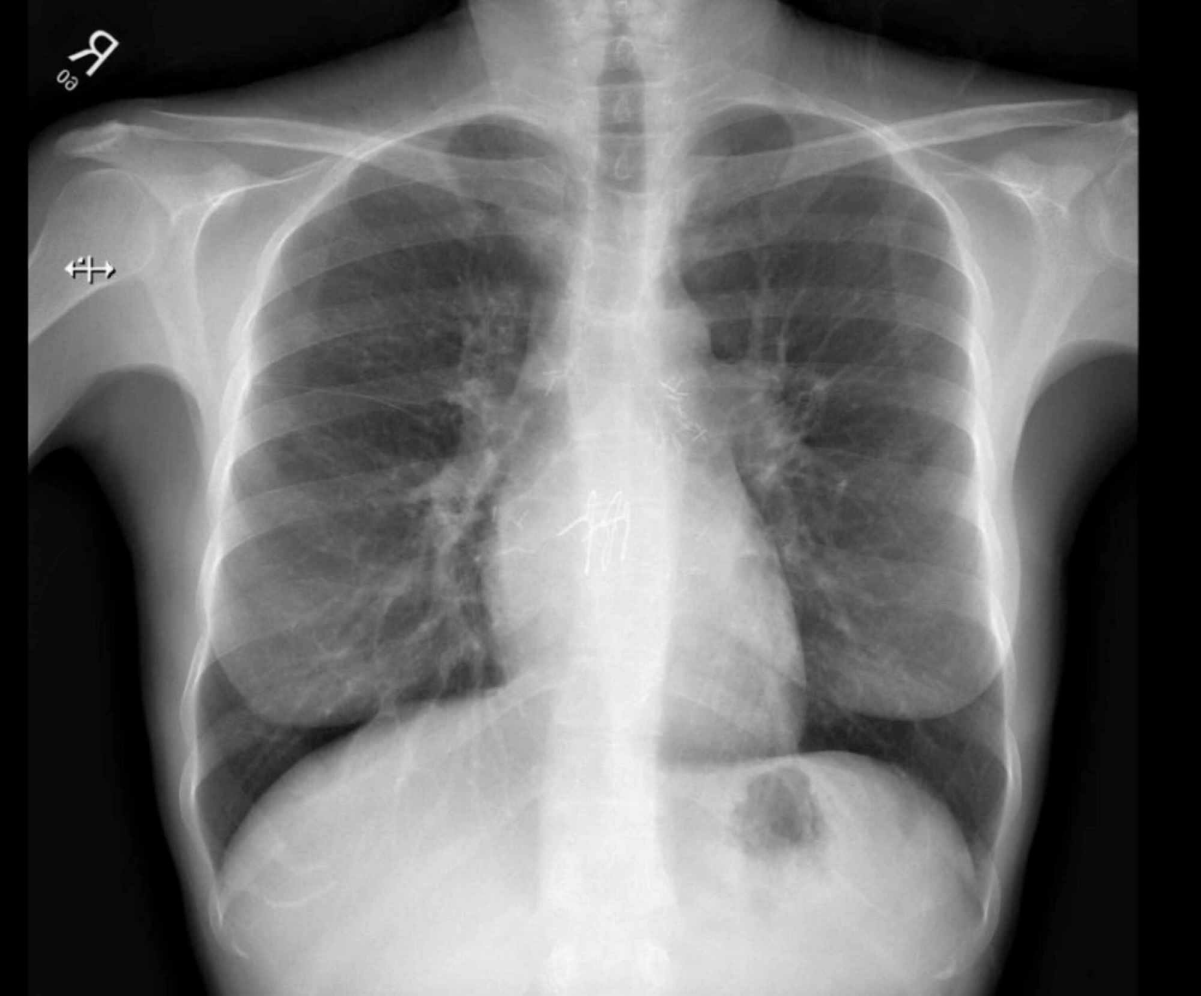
Chest X-ray six weeks after therapy.
